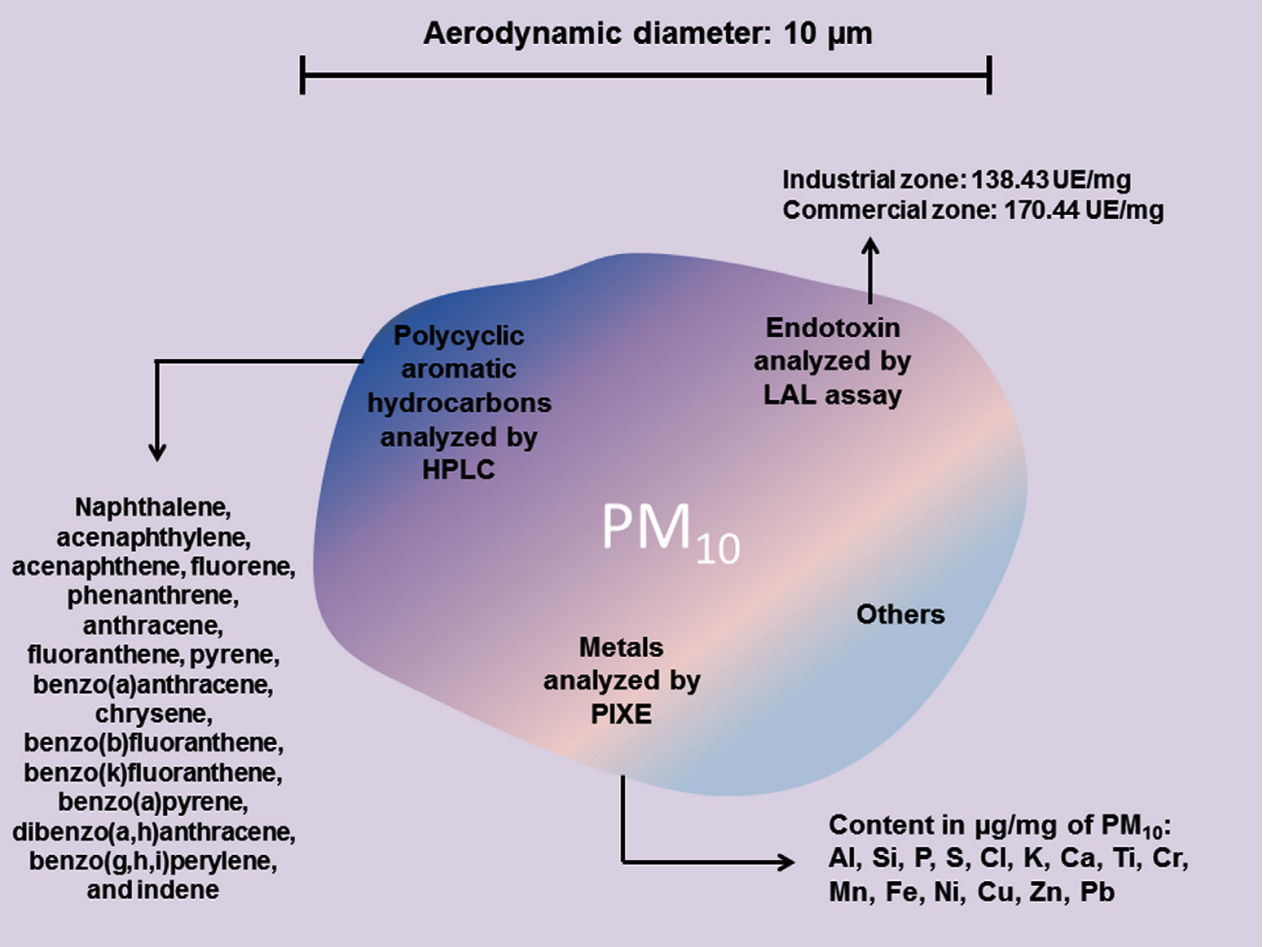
The composition of particulate matter with aerodynamic diameter of 10 μm (PM_10_). PM_10_ is a complex mixture of polycyclic aromatic hydrocarbons (PAHs), metals and endotoxin, among others. PAHs were analyzed by high-pressure liquid chromatography (HPLC); metals by particle-induced X-ray emission (PIXE) analysis, and endotoxin content was analyzed by limulus amebocyte lysate (LAL) assay.
